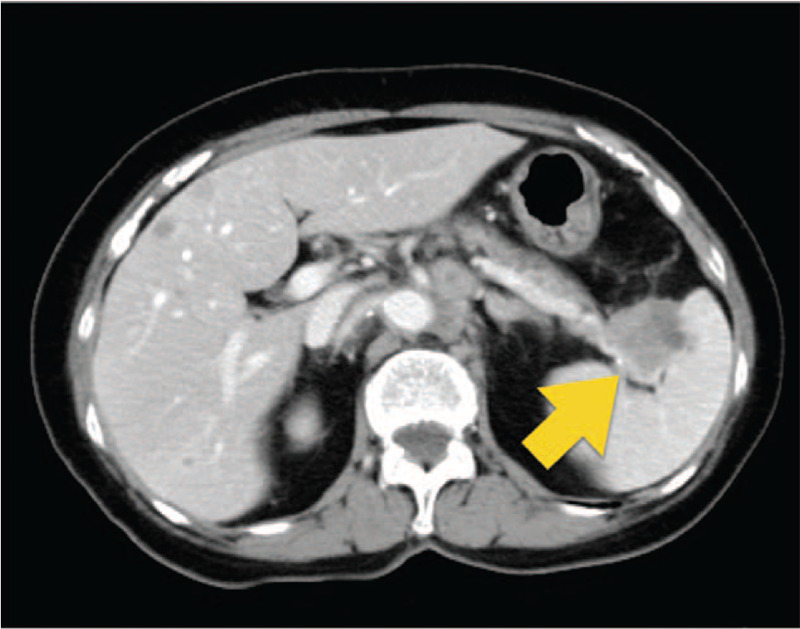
Abdominal computed tomography scan showing obstruction of the splenic vein by the tumor in case 2.
